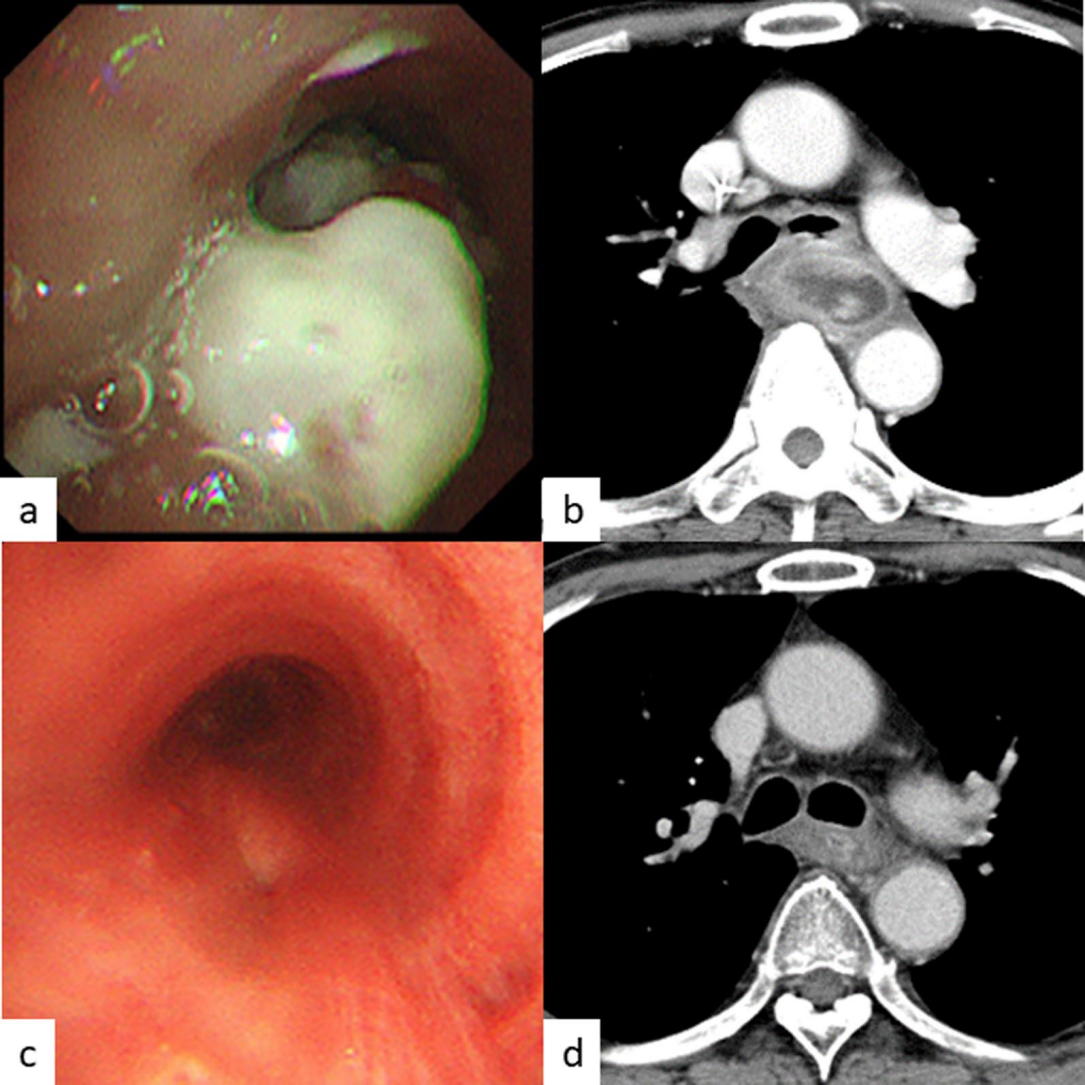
Images from bronchoscopy and CT. a Before CRT, bronchoscopy shows a white plaque-like erosion at the left bronchus. b Before CRT, CT shows the tumor invading the left bronchus. c After CRT, bronchoscopy shows a slightly depressed lesion. d After CRT, CT shows that the tumor has become smaller. There is no evidence of tumor invasion of the bronchus
